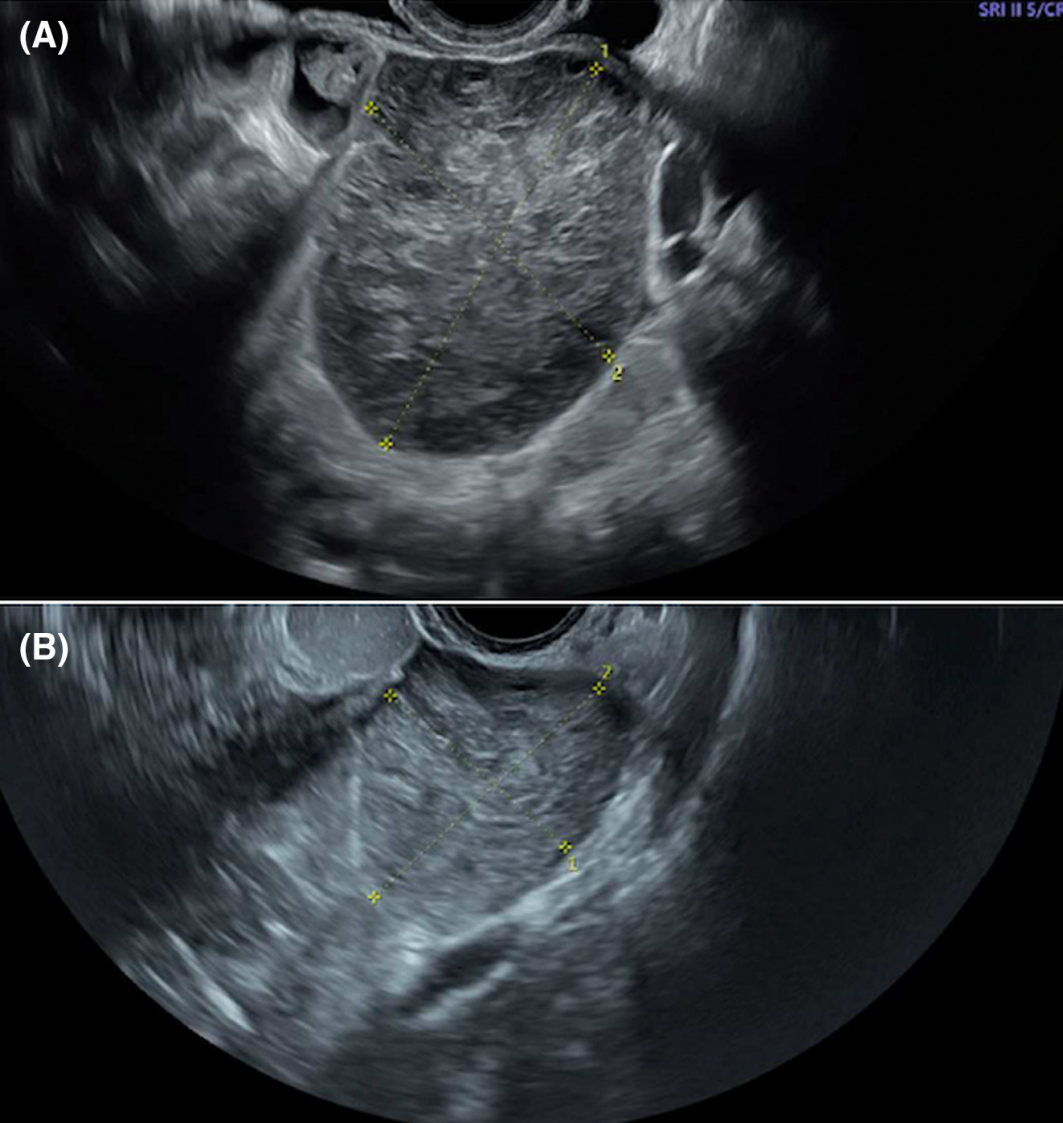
Dimensions at ultrasound of case 1 before (A) and after (B) treatment with drospirenone.
